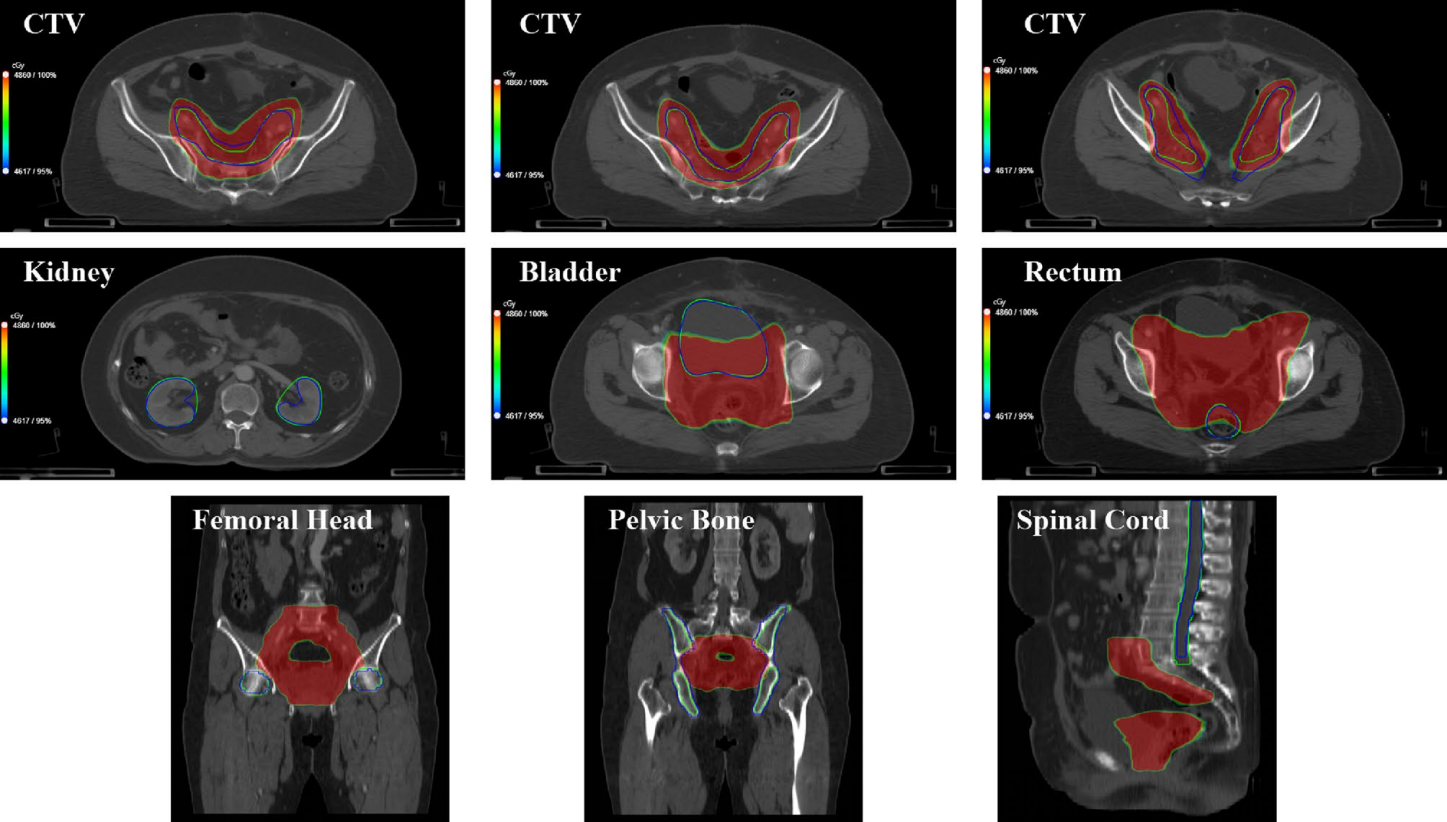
Results of delineations and dose distributions for CTV and OARs in CT slices. The green lines represent manual contours approved by the senior physician; the blue lines represent DL based contours; colourwash represent dose distributions with the range of 95% prescription to 100% prescription.
